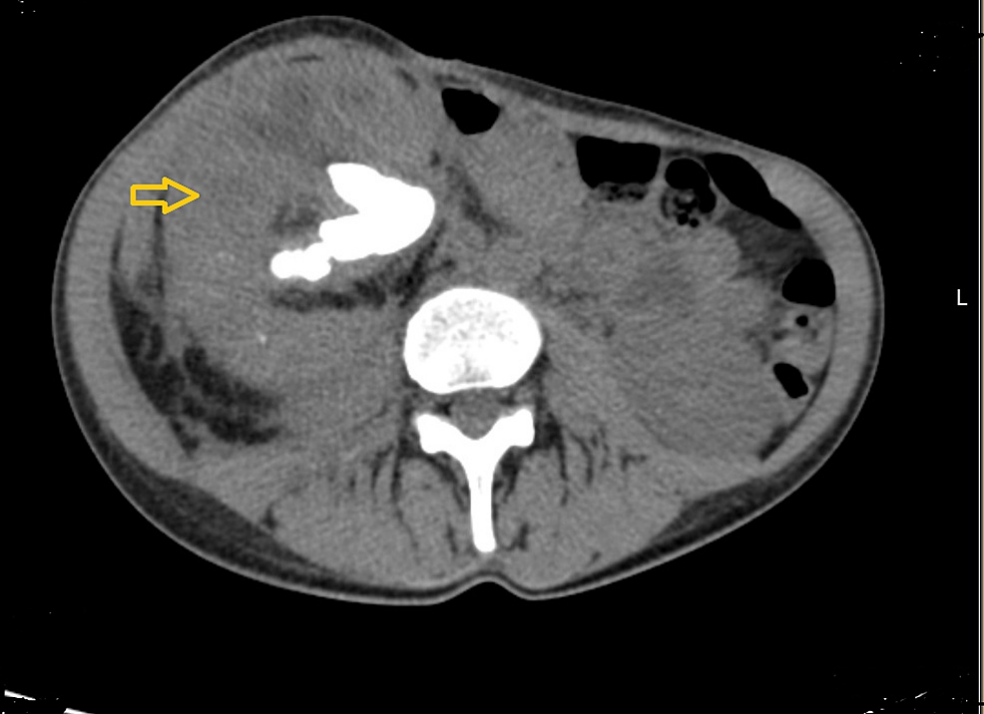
Abdominal and pelvic CT scan demonstrating kidney enlargement with calyceal dilatation
